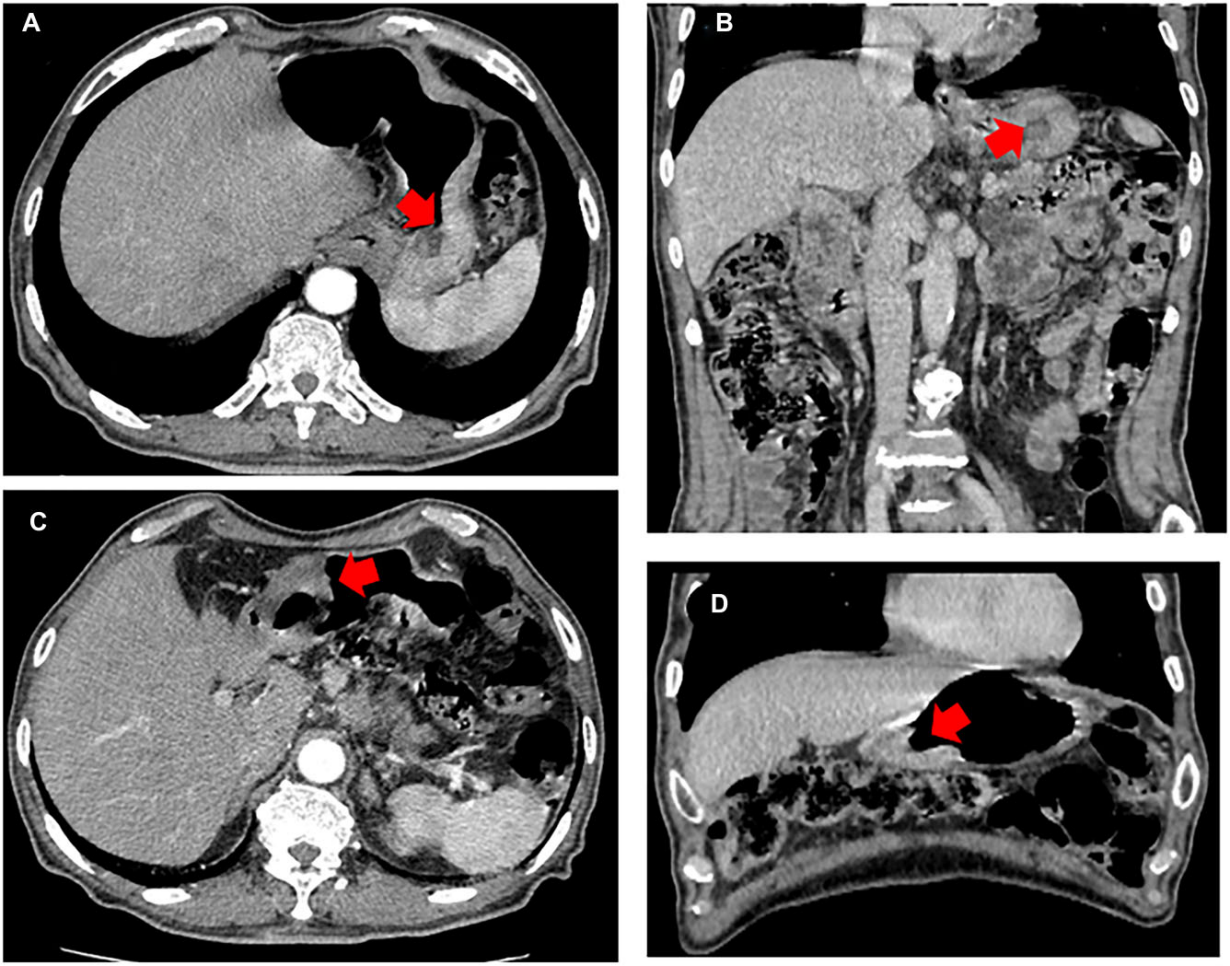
Preoperative abdominal computed tomography (CT) for gastric cancer. (A) Transverse section of the tumor located at the greater curvature of the upper stomach. (B) Coronal section of the tumor located at the greater curvature of the upper stomach. (C) Transverse section of the tumor located at the antrum of the stomach. (D) Coronal section of the tumor located at the antrum of the stomach. The red arrow represents the tumor location. (A colour version of this figure appears in the online version of this article.)
